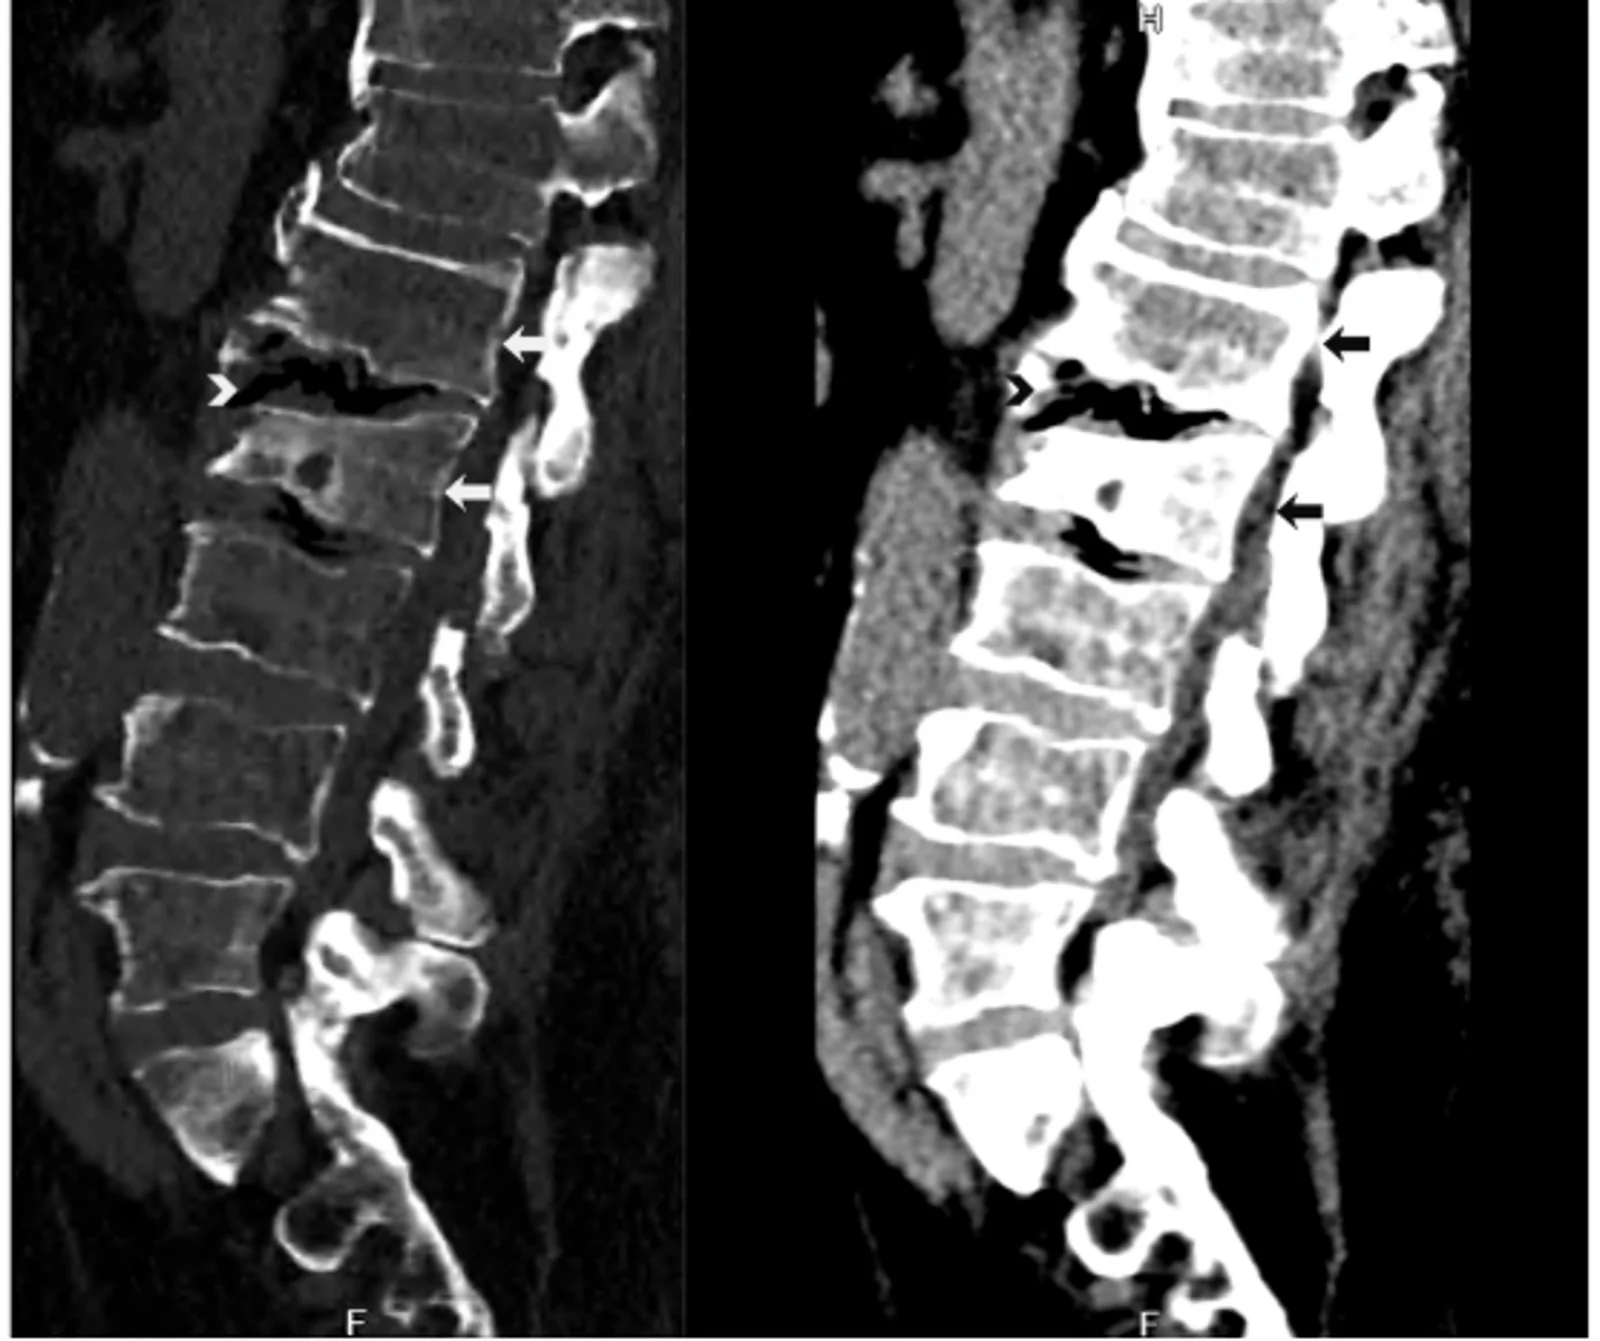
(A) CT performed at admission. Arrowhead indicates vacuum phenomenon in the disc space. Long arrows indicate vertebrae L1 and L2
